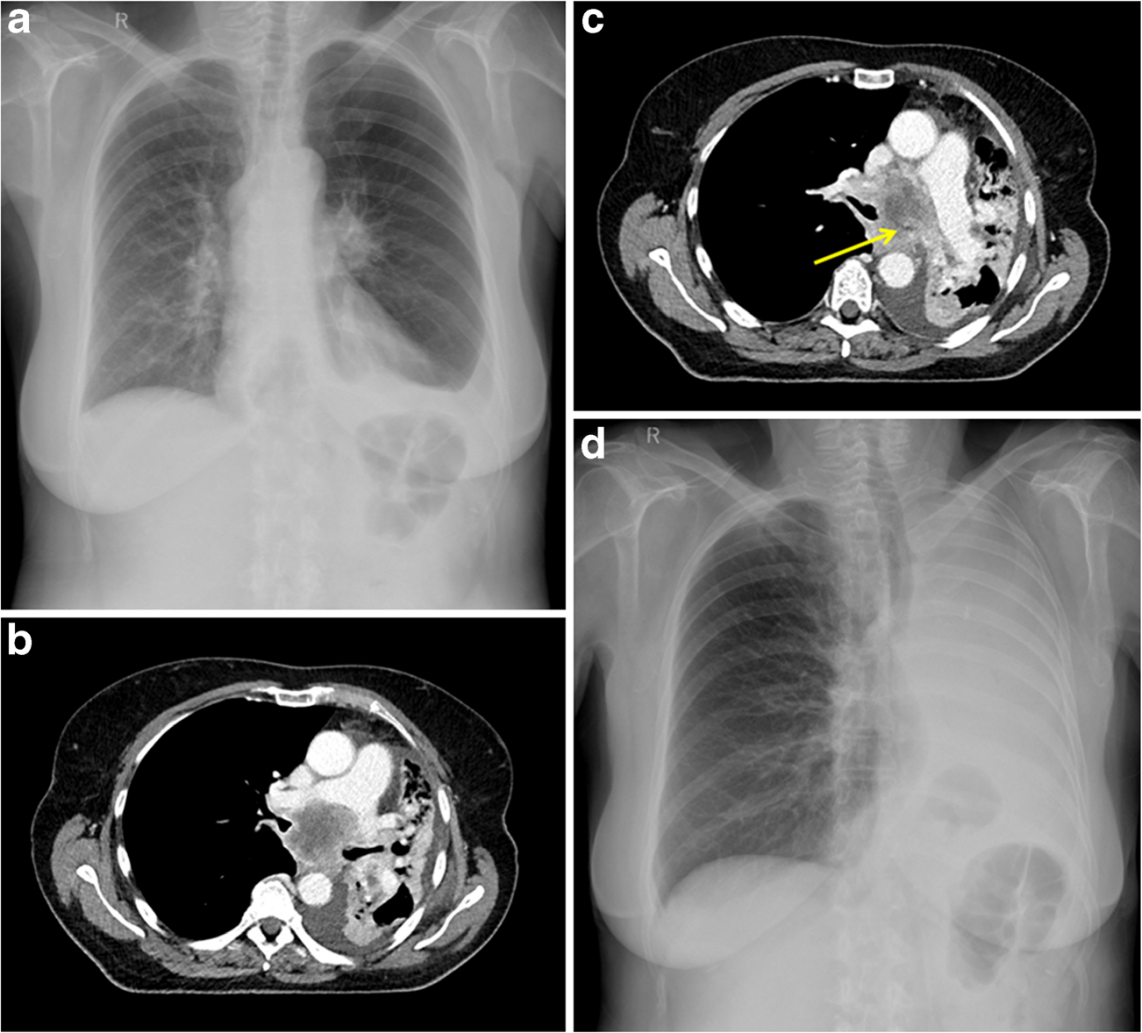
A 64-year-old woman was referred to the pulmonologist for persistent cough and increasing dyspnea. a Erect postero-anterior chest radiograph at the time of presentation shows an enlargement of the left hilum, air trapping in the left hemithorax (suggestive of partial bronchial obstruction) and a left-sided pleural effusion. b Axial contrast-enhanced chest CT in mediastinal window 1 day after the chest radiograph shows a left hilar mass with infracarinal extension, c partially obstructing the left main bronchus (*yellow arrow*). Although there is only a short time frame between the radiograph and the CT, there is marked volume loss of the left lung and mediastinal shift caused by retro-obstructive atelectasis. Diagnosis of stage IV non-small cell lung cancer was made. Four days after the CT examination, the patient experienced an acute episode of severe dyspnea and stridor for which she was referred to the emergency department. d Erect postero-anterior chest radiograph at admission shows a white lung on the left with massive mediastinal and cardiac shift, caused by complete obstruction of the left main bronchus. No air can be delineated in the left main bronchus. This was confirmed by bronchoscopy
